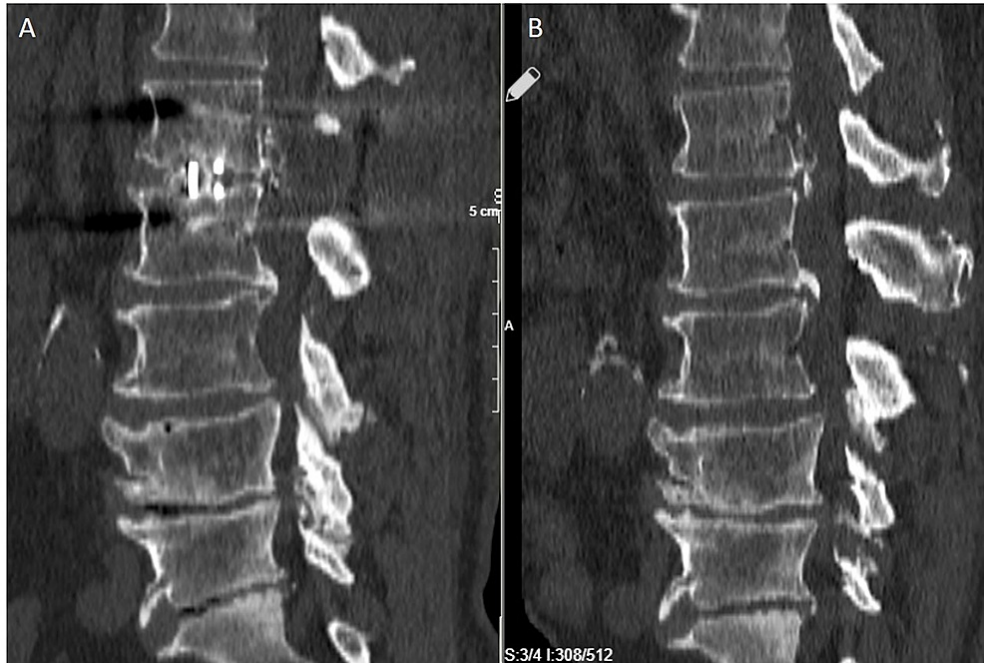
A: Postoperative 2nd Year CT, B: Preoperative CT
